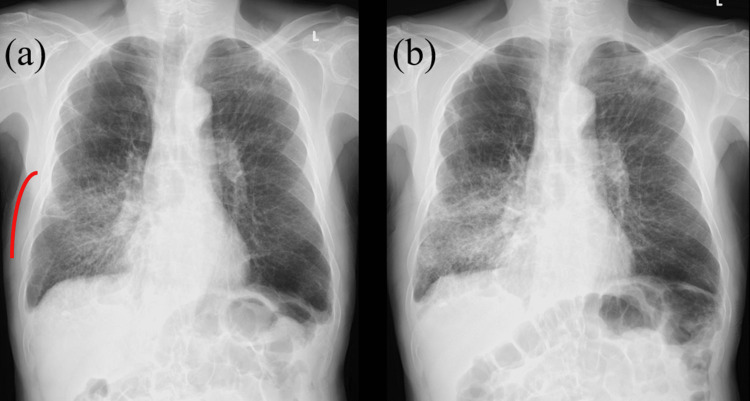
X-ray photographs acquired (a) two weeks (RP marked by a red line) and (b) three weeks after terminating radiotherapy, the latter showing more significant RP RP - radiation pneumonitis
